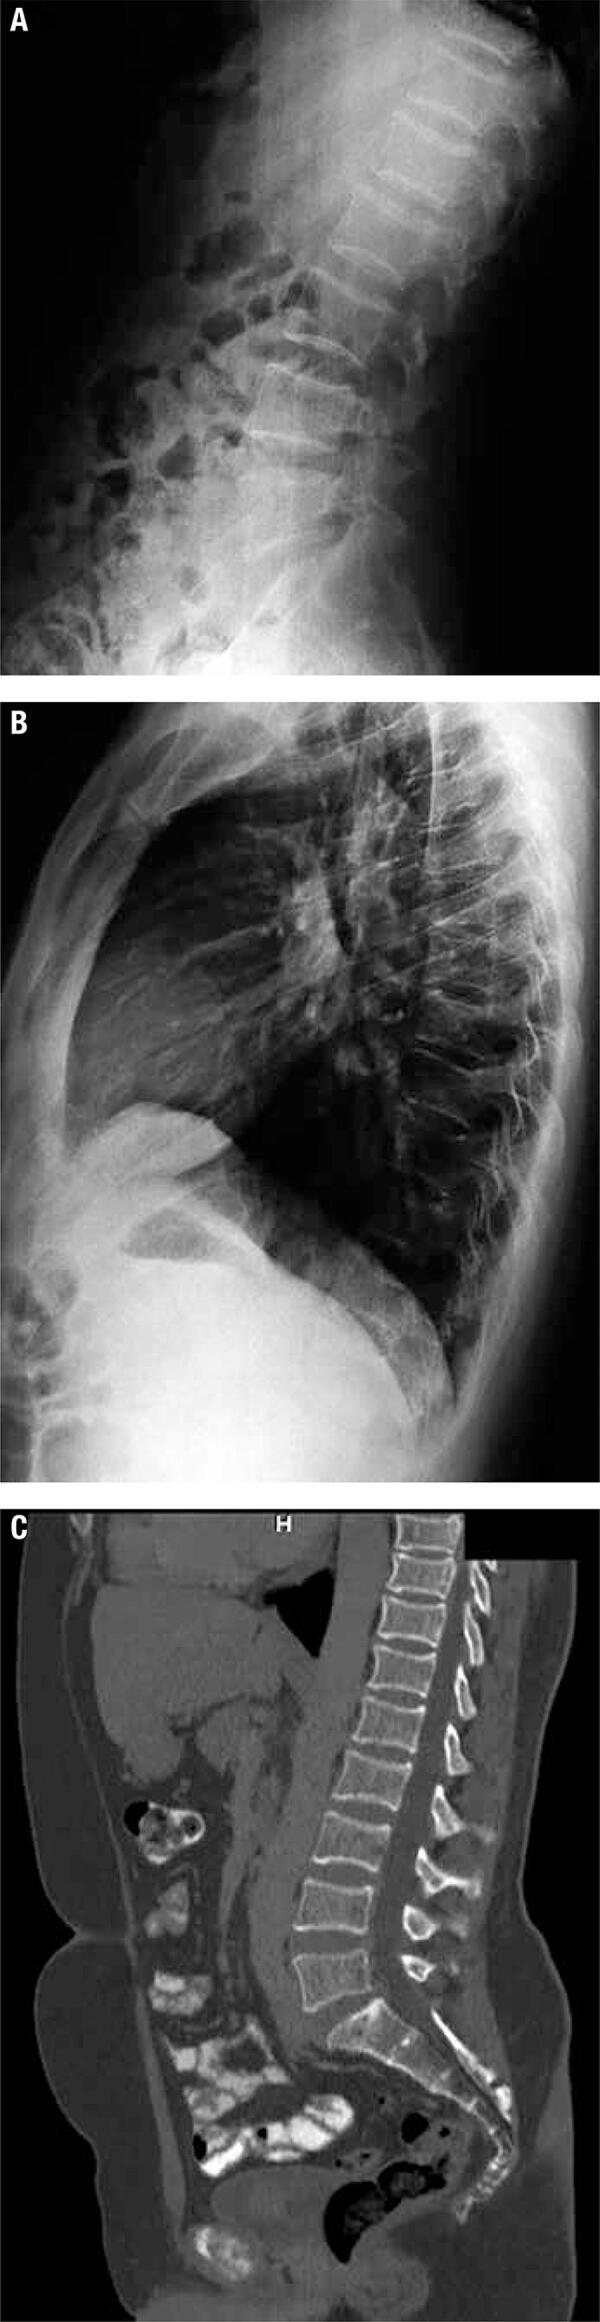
Vertebral fractures (A+B+C). (A) Profile lumbar X-Ray. (B) Profile thoracic X-Ray. (C) Computed tomogaphy image of the spine.
